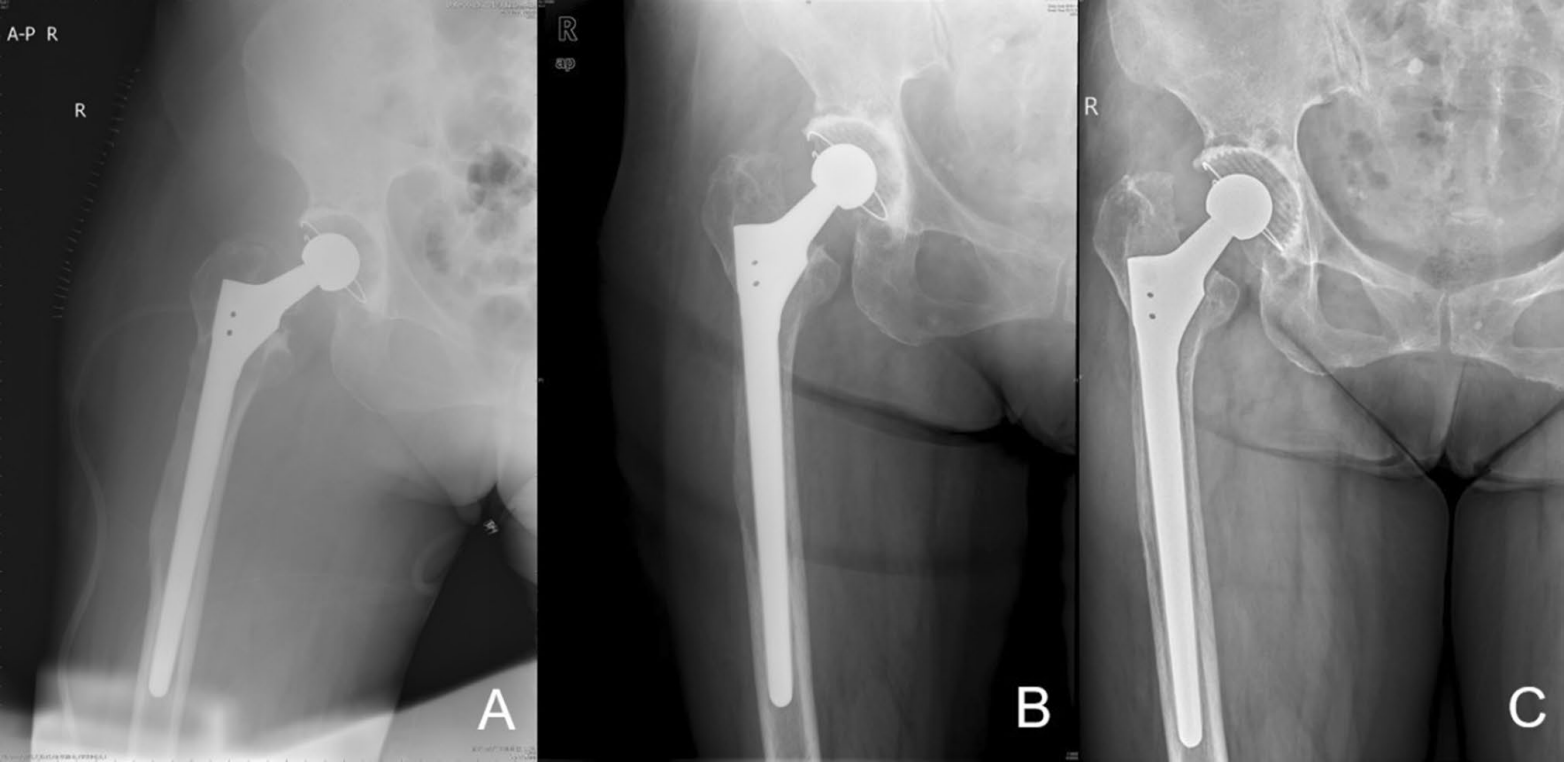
Postoperative radiographs of high-grade femoral defect managed with a nonmodular stem with stem subsidence.
